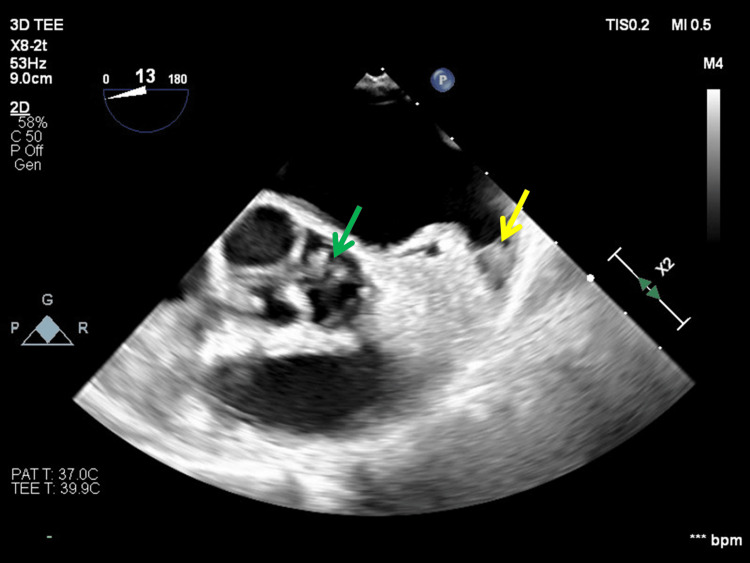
Transesophageal echocardiography showing mass lesions within the left atrial appendage (yellow arrow) and the aortic valve (green arrow).
